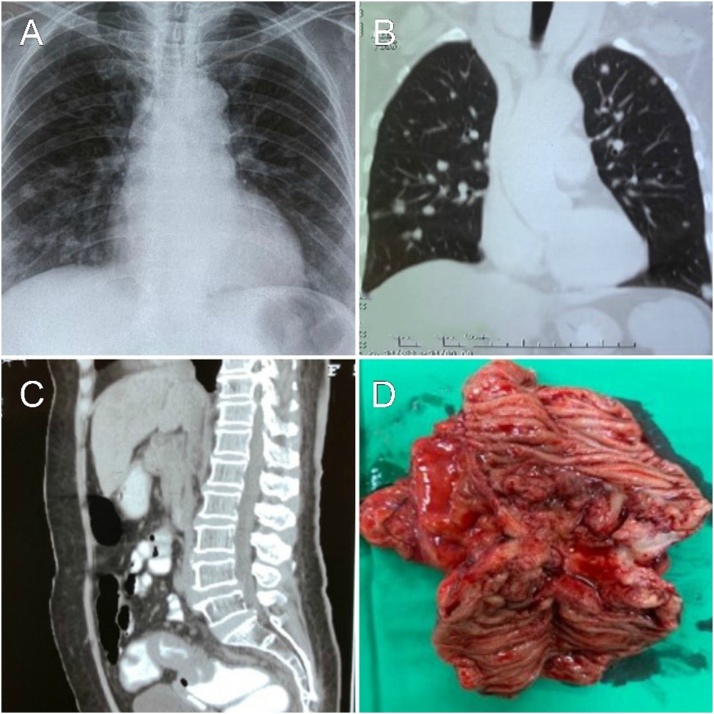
A) Chest X-ray showed multiple metastatic nodules, B) Chest MSCT showed multiple metastatic nodules, C) Abdominal MSCT showed sigmoid tumour, and D) Intraoperative finding of rectosigmoid tumor.
